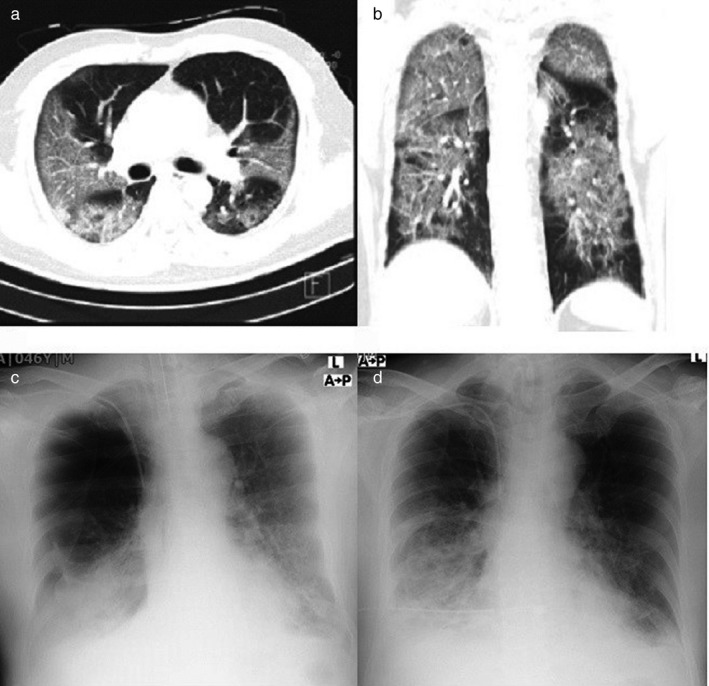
(a b) Coronal and axial slices of chest CT scan on admission showed ground‐glass opacity and multifocal crazy paving patterns involving both lungs, predominantly in peripheral, and paraseptal emphysema on first left lung segment; (c) chest X‐ray on April 10 showed right lung hydropneumothorax and atelectasis, bilateral lung opacities on perihilar and middle to inferior lung fields, cardiothoracic ratio 54% with elongation and calcification of aorta, ETT, and CVP; (d) chest X‐ray post first bronchoscopy, water seal drainage (WSD), and tracheostomy on April 11: Remaining pneumothorax on superior region of right lung, WSD installed with distal tip as high as 10th thoracic vertebra on right lung side
